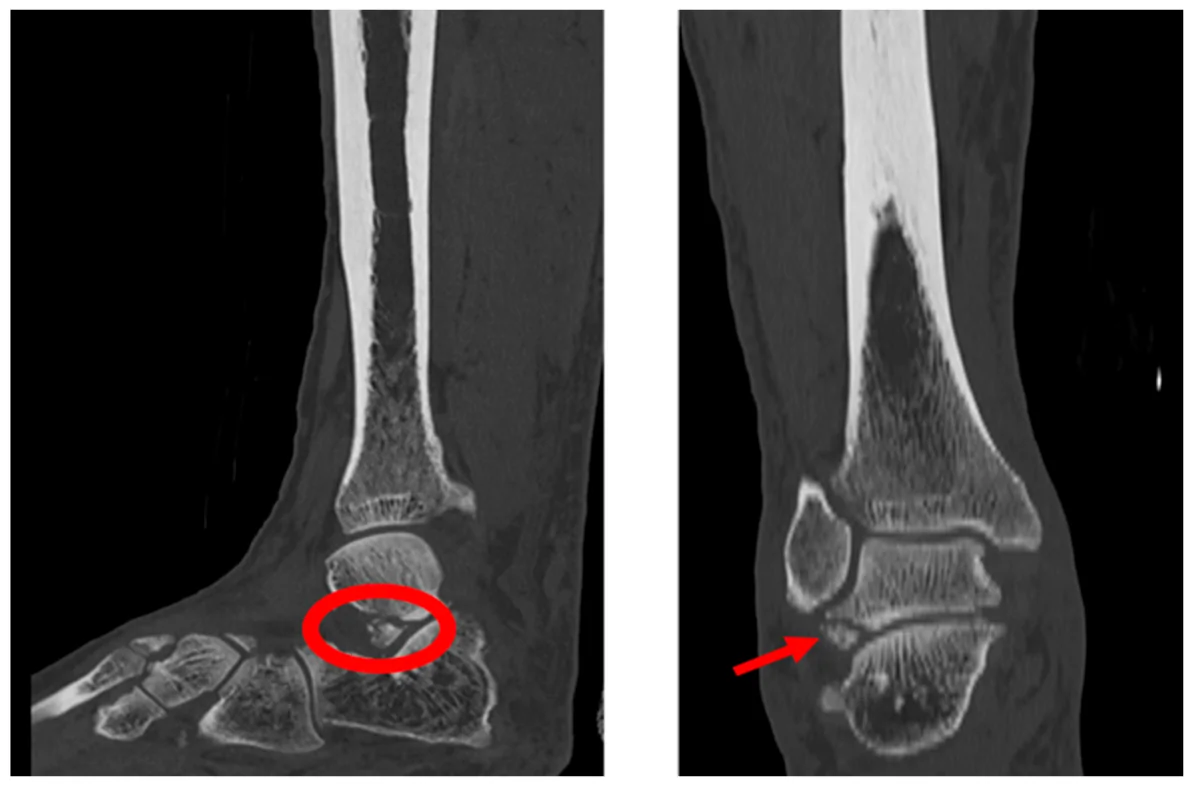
Computed tomography (CT) sagittal and coronal cross-section. The applied cast has been removed for imaging purposes. Red circle and arrow indicate the fracture.
